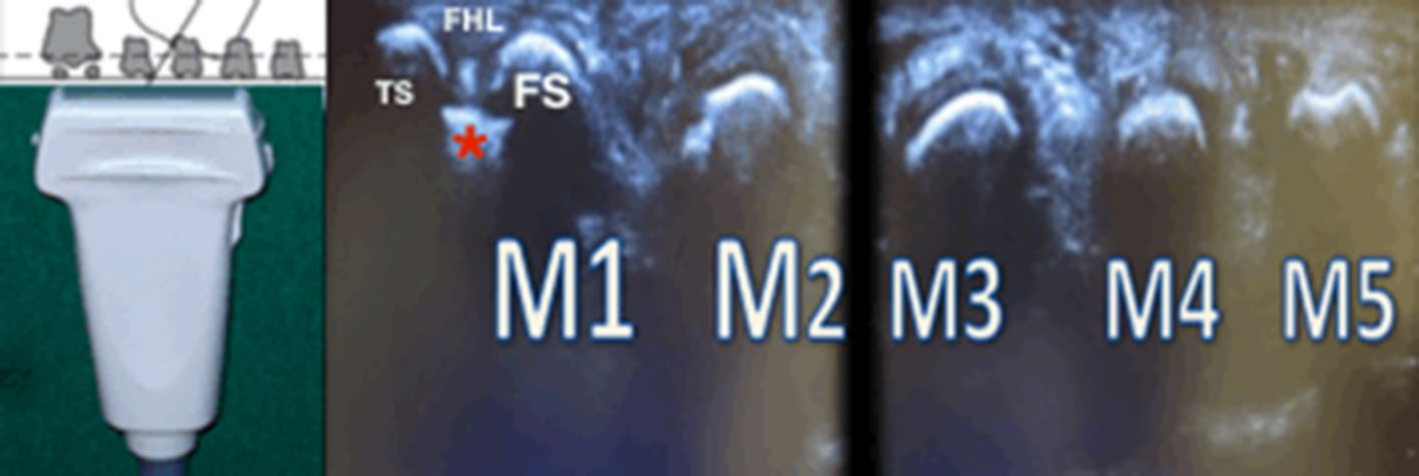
Scheme with ultrasonic probe showing the sesamoid bones and the first-fifth metatarsal heads (M1–M5). Note the tibial sesamoid (TS), fibular sesamoid (FS) centered on the crest of M1 (red asterisk) and the flexor hallucis longus (FHL) positioned between both sesamoid bones
